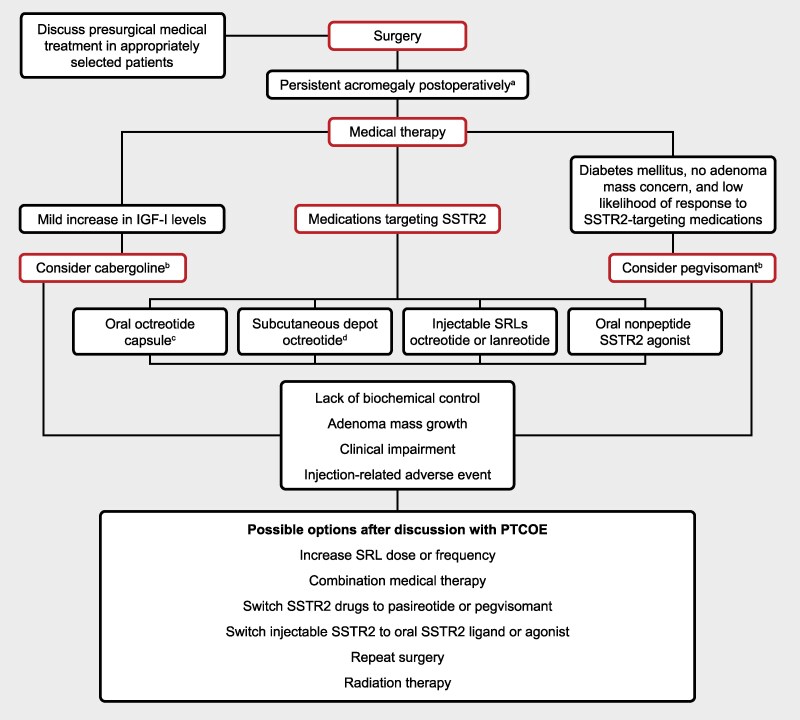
Treatment landscape in acromegaly. ^a^Consider reoperation in selected cases with large surgically-accessible residual adenomas. ^b^Indication as a first-line treatment is not approved in all countries; cabergoline is not FDA-approved for acromegaly. ^c^FDA approved for responders to injectable octreotide LAR and lanreotide depot. ^d^Not approved in all countries. Possible options after discussion with PTCOE: Persistent adenoma growth may raise the concern of an aggressive adenoma. Treatment options should be discussed in multidisciplinary tumor boards to include surgery, radiation techniques, addition of SRLs to pegvisomant monotherapy, or switch from octreotide or lanreotide to pasireotide or combination of pasireotide to pegvisomant. Close MRI monitoring should be performed. Adapted from Melmed et al Consensus on acromegaly therapeutic outcomes: an update. *Nat Rev Endocrinol*. 2025;21(11):718-737 with permission from Springer Nature. Abbreviations: IGF-I, insulin-like growth factor 1; PTCOE, Pituitary Tumor Centers of Excellence; SRL, somatostatin receptor ligand; SSTR2, somatostatin receptor subtype 2.
